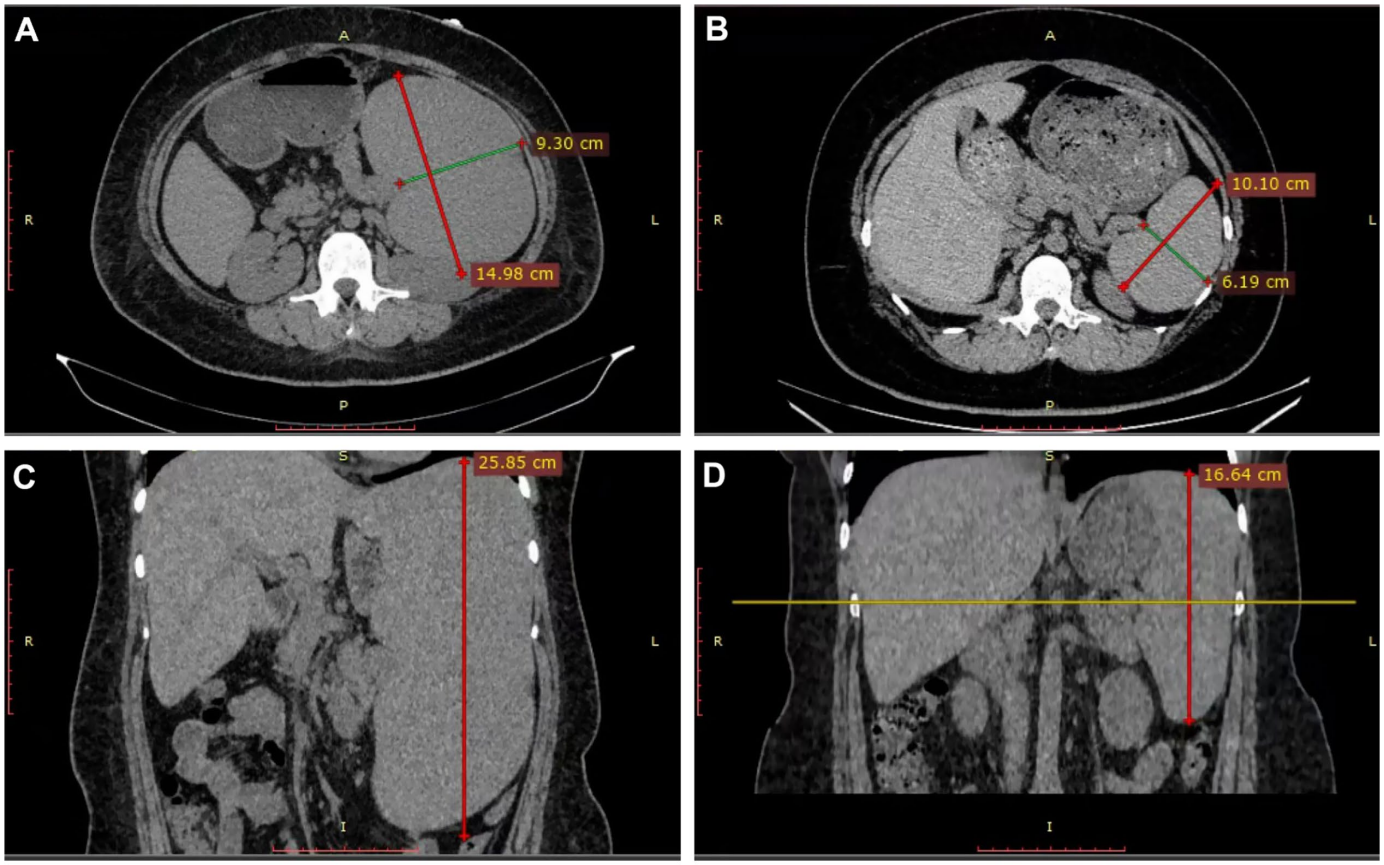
Non-contrast CT before (A,C) and 4 months (B,D) after liposomal amphotericin B therapy. Measurements were made at the level of splenic hilum.
